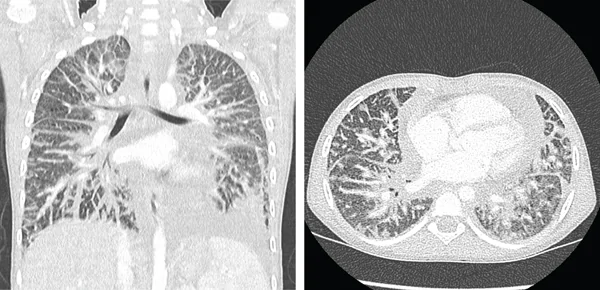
CT scan axial and coronal images showing the main pattern of ILD presented by the patient.
